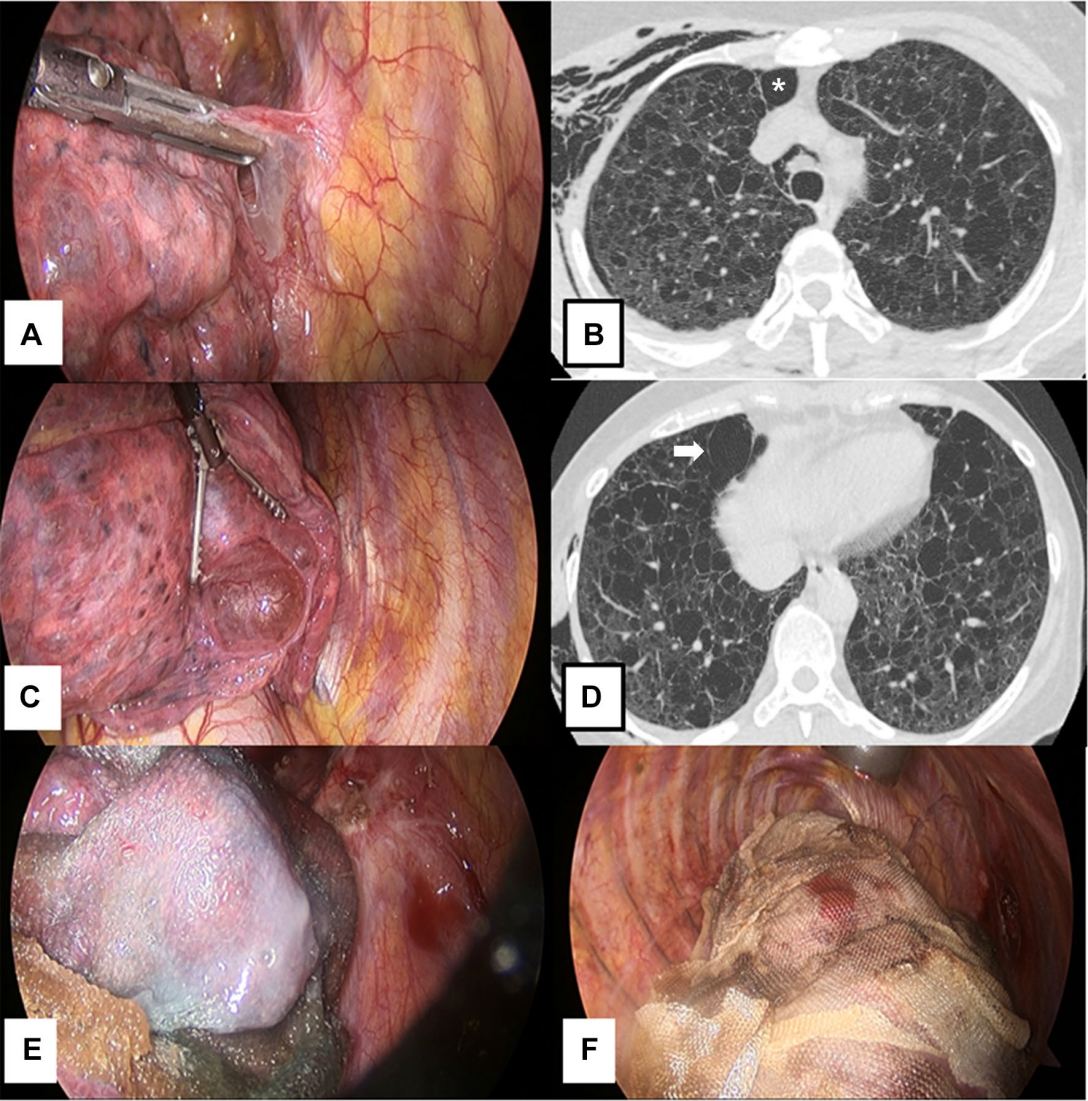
Gross findings of lungs correlated to computed tomographic images. a Ruptured bullae were evident in the right upper lobe, as was a dense adhesion around the bullae. The adhesion was released and the bullae loop ligated. b The ruptured area (asterisk) contained bullae, as revealed by CT. c A huge fragile cyst at risk of immediate rupture was covered by an absorbable polyglycolic acid sheet (e). d The fragile cyst (arrow) was located near the bullae, as revealed by CT. f We performed total pleural coverage (TPC) of the entire lung
